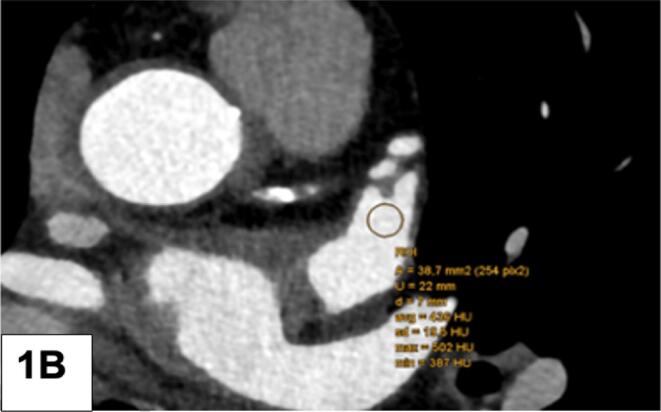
A LAA CT density (HU) quantification: 3 regions of interest (ROI) were placed from LAA tip to the bottom and the gradient was calculated. A normal LAA, entirely filled with contrast agent, is shown. B: Mean LAA HU density of a normal LAA: A large ROI was placed into the LAA.
